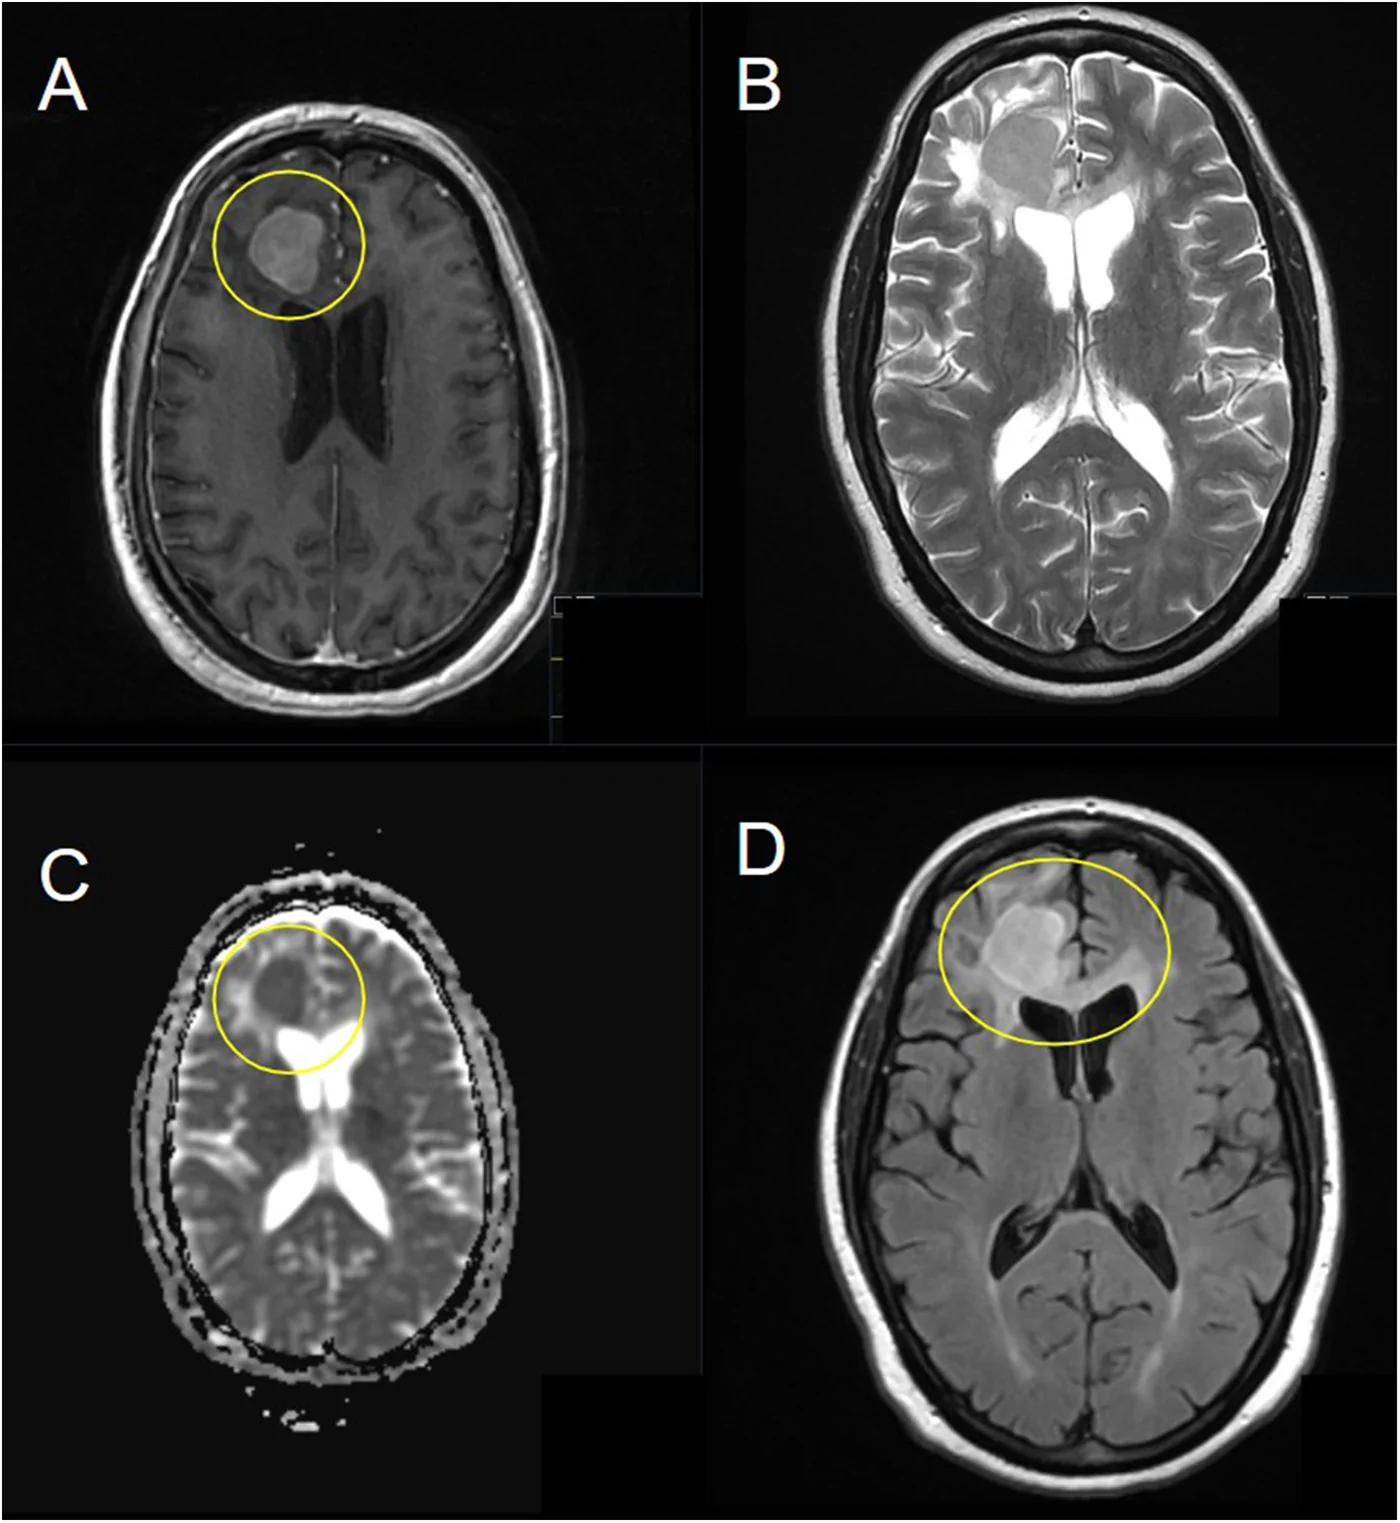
Primary central nervous system lymphoma. (A) Axial T1-weighted post-contrast image demonstrates a homogeneously enhancing solid lesion in the right frontal lobe extending into the genu of the corpus callosum without gross internal necrosis (yellow circle). (B) Axial T2-weighted image shows a hyperintense mass. Hyperintense signal extends along the genu to the contralateral side, likely representing reactive edema. Mild to moderate surrounding vasogenic edema is present without midline shift or herniation. (C) Apparent diffusion coefficient map demonstrates reduced ADC values within the lesion (yellow circle), suggesting high cellularity and supporting a higher-grade neoplasm. (D) Axial FLAIR image demonstrates a homogeneously hyperintense lesion with edema extending across the genu of the corpus callosum (yellow circle) and associated perilesional edema.
